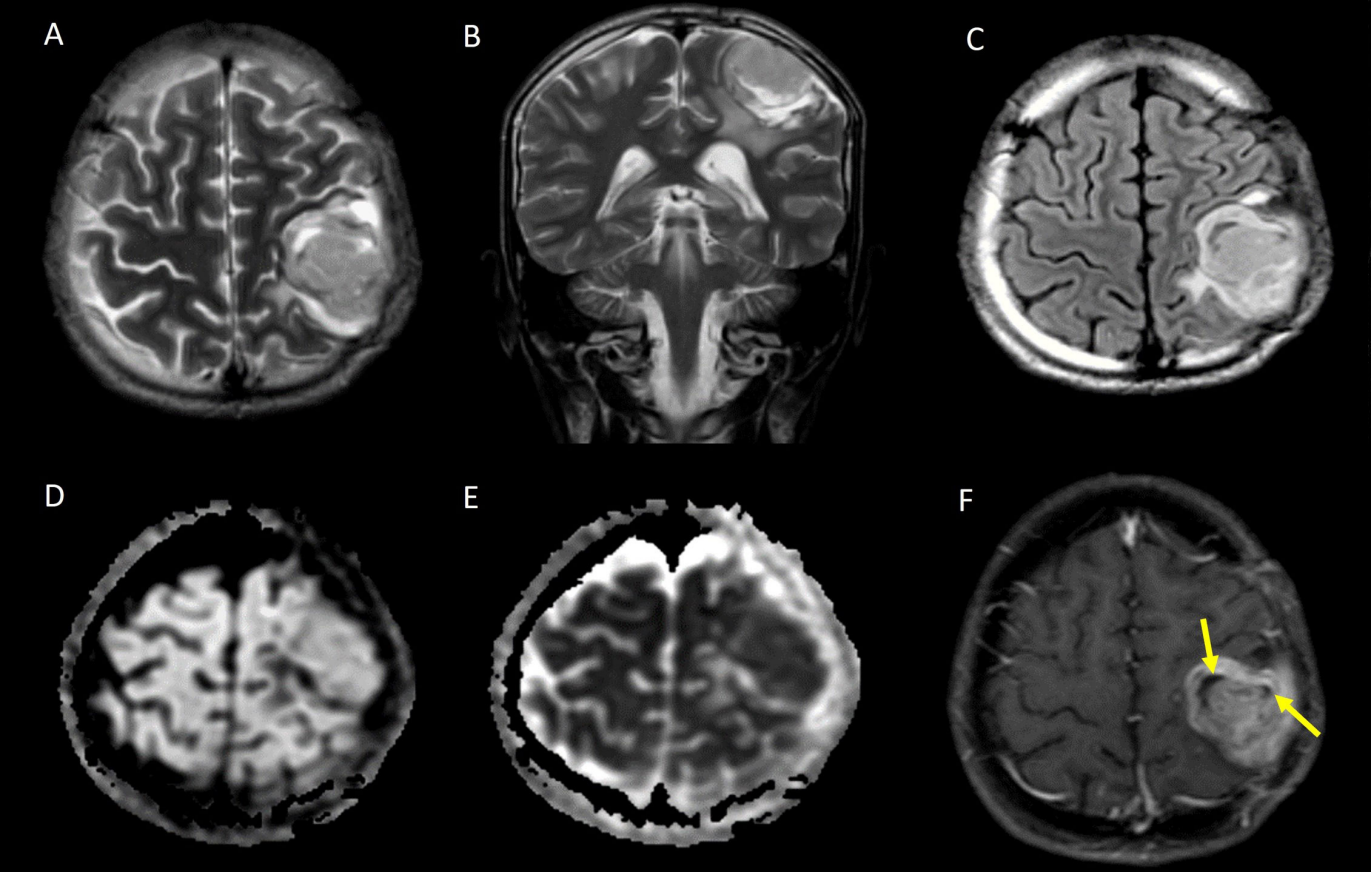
Cortical based STE. A. Axial T2W image and B. coronal T2W image showing a heterogeneously hyperintense solid mass with few cystic areas in the cortex of the left frontal lobe; C. axial T2 FLAIR image shows the cystic areas are not suppressed; D. the lesion appears isointense on diffusion-weighted imaging (DWI); E. it shows low apparent diffusion coefficient (ADC) values suggestive of restricted diffusion; F. axial contrast-enhanced T1W image showing heterogeneous post contrast enhancement, with the cystic areas corresponding to non-enhancing areas of necrosis (yellow arrows)
